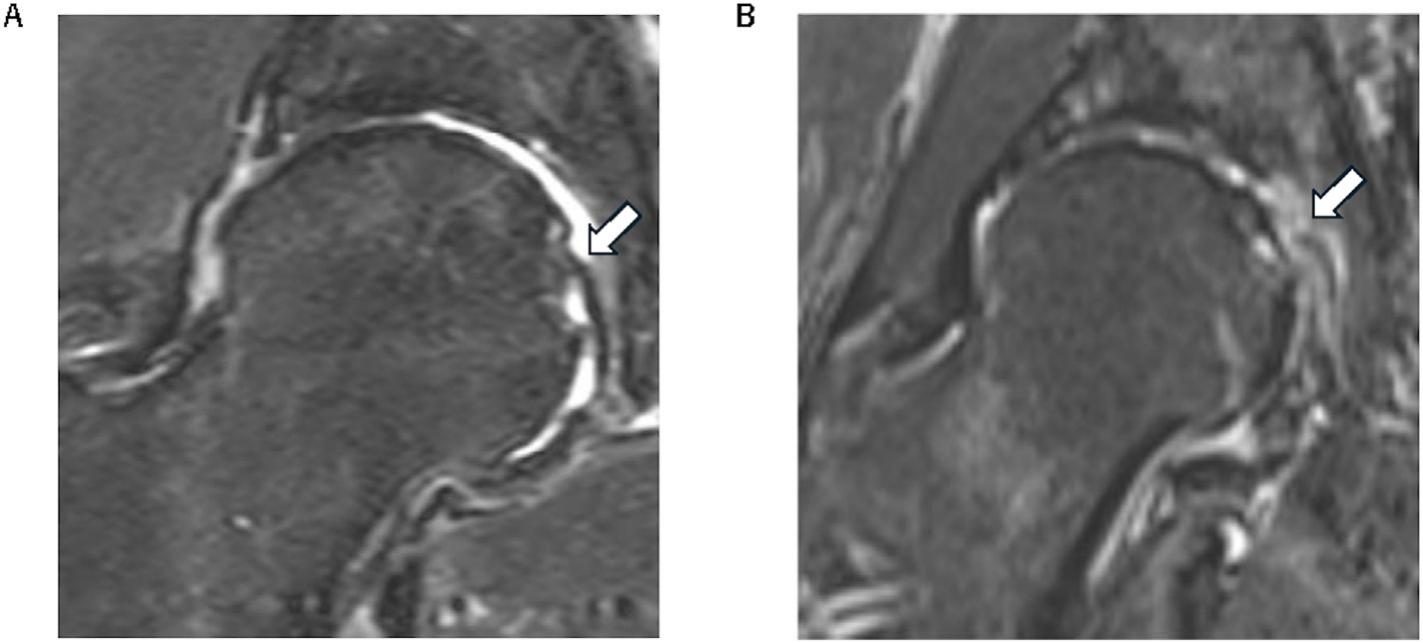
Ligamentum teres on coronal T2-weighted fat-suppressed images. (A) Representative image of the ligamentum teres (arrow) without evidence of tear. (B) Representative image of torn ligamentum teres. The arrow indicates discontinuity of the ligament.
